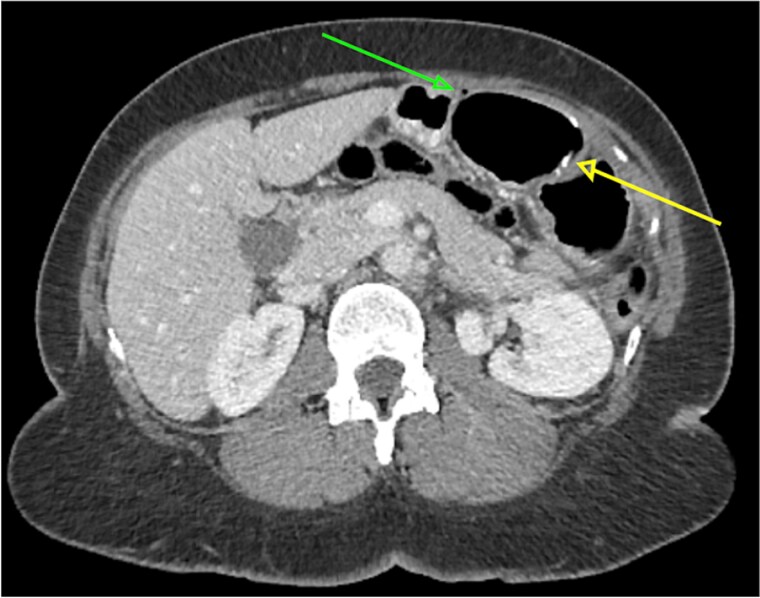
Axial abdominal CT scan showing a 4 cm hake fishbone (lower arrow) and free intraperitoneal air in the abdominal cavity (upper arrow).
